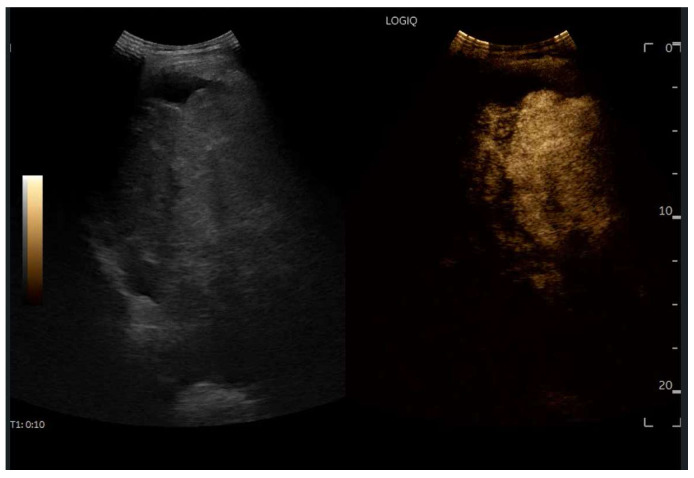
Contrast-enhanced ultrasound (CEUS) in the arterial phase; note the heterogeneous wash-in of multiple hepatic areas.
